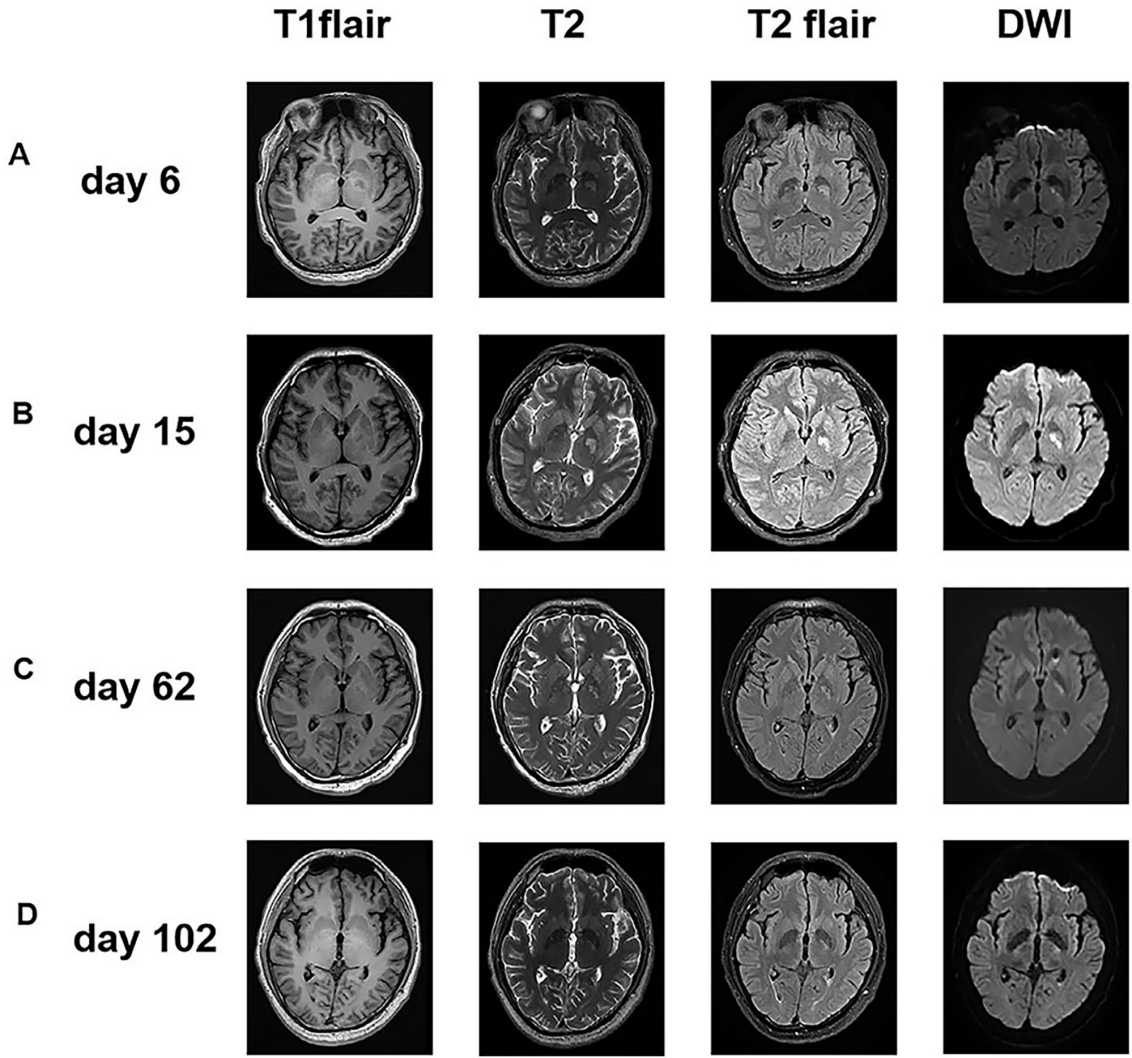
Serial axial brain MR imaging of the patient. (A) Imaging on Day 6 showed abnormal enhancement in left basal ganglia(T2/T2 Flair/DWI). (B) The imaging on Day 15 reveals the persistence of a lesion in the patient’s left basal ganglia prior to the omaya capsulotomy procedure. Furthermore, the images indicate that there are no contraindications to surgery in this patient. (C) Imaging on Day 62 showed marked improvement after effective antifungal therapy. The atypical signaling observed in the left basal ganglia is characterized by a notable reduction. (D) Imaging on Day 102 showed no marked lesion after corticosteroid treatment.
